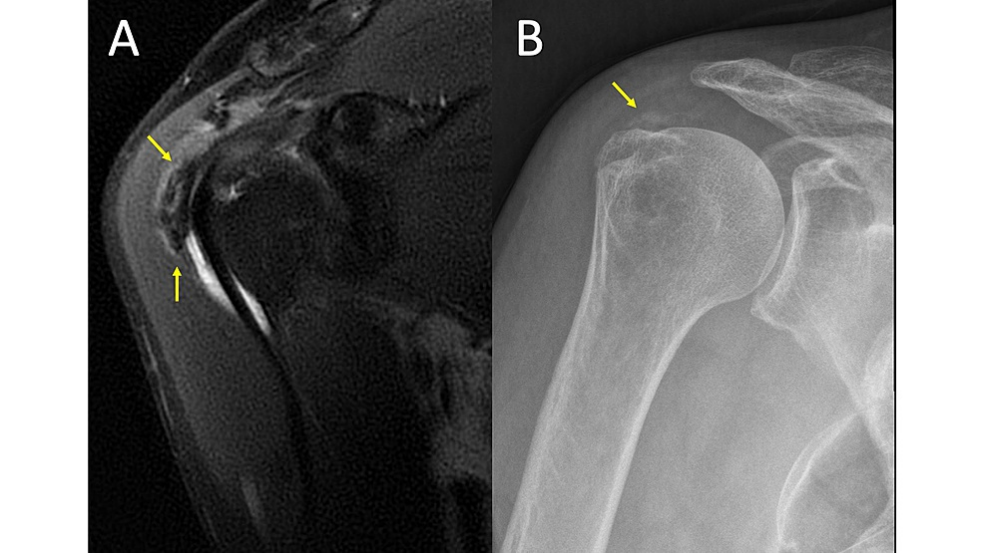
Hydroxyapatite Deposition Disease MRI coronal T2 fat saturation (FS) (1A) and plain radiograph of the shoulder in the Grashey view (1B) demonstrate mineralization within the soft tissues adjacent to the greater tuberosity (arrows). While easy to appreciate on the MRI in this case because of the extent of calcification and interval rupture into the bursa (MRI obtained several months after the radiographs), HADD can be difficult to distinguish from surrounding tendons and easily overlooked on MRI without comparison radiographs.
